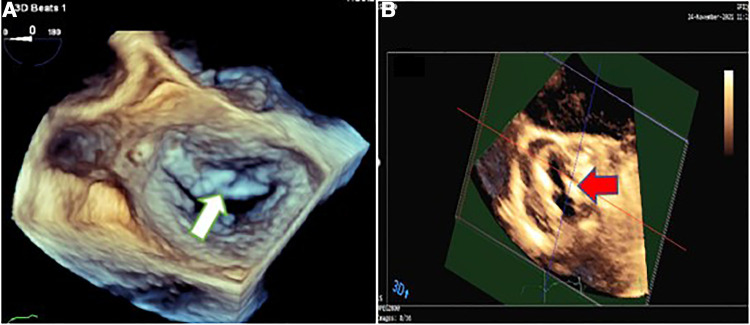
Three-dimensional transesophageal echocardiography showing mitral valve endocarditis with irregular thickening over the atrial side of the base of anterior mitral valve leaflet (white arrow) (A) and irregularly thickened and destructed bicuspid aortic valve involved by infective endocarditis (red arrow) (B).
